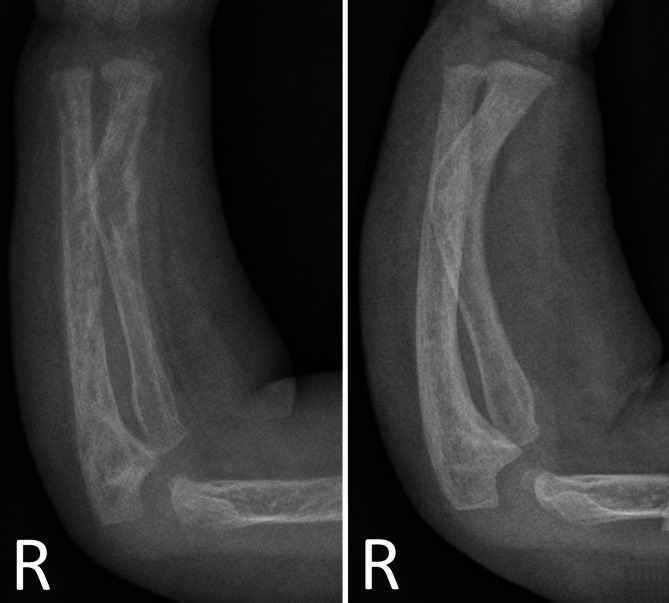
X-ray follow-up of right upper extremity, before and after trametinib therapy
